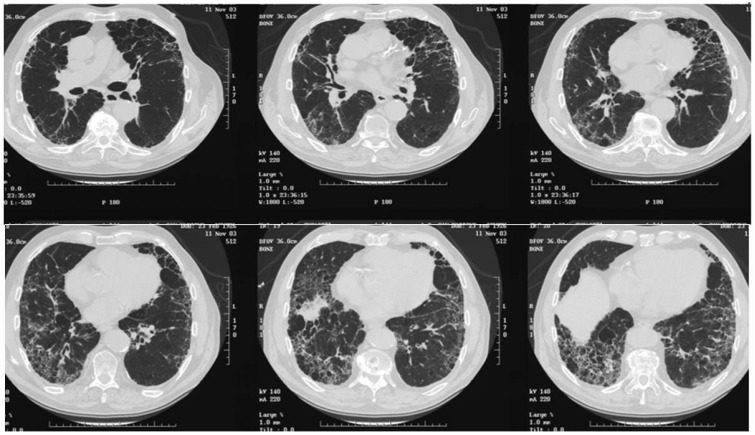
Series of chest HRCT images showing IPF characterized by reticular pattern and honeycombing associated with pulmonary emphysema.
